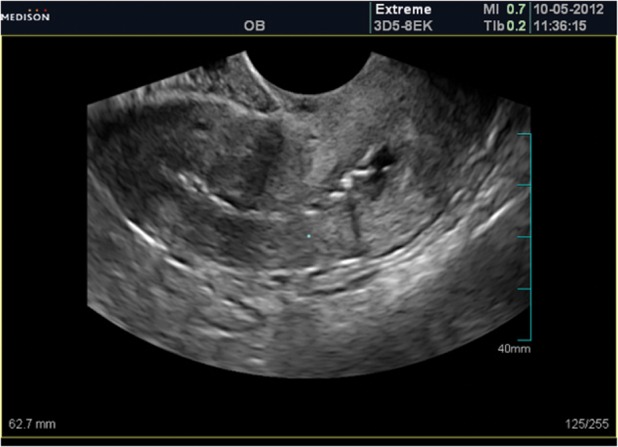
Ultrasound shows irregular endometrial contour
